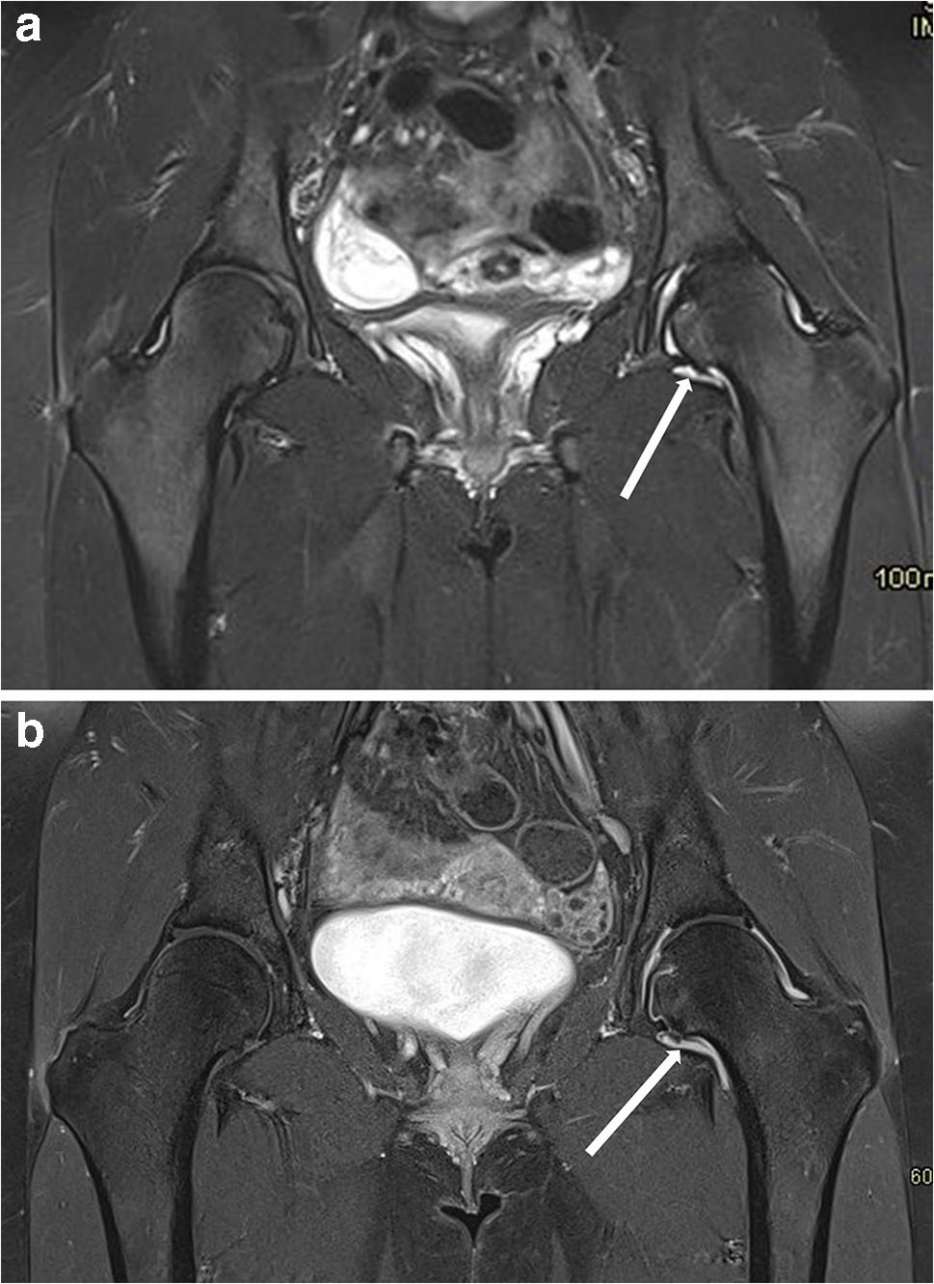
Coronal magnetic resonance imaging of the hips in a 15-year-old girl with psoriatic arthritis (HLA B27 negative, ANA positive). a T2 turbo inversion recovery image shows an effusion in the left hip joint (*arrow*); bone and cartilage are normal in appearance. b Postcontrast T1-weighted fat-suppressed image shows synovial enhancement on the left (*arrow*)—a sign of active synovitis
